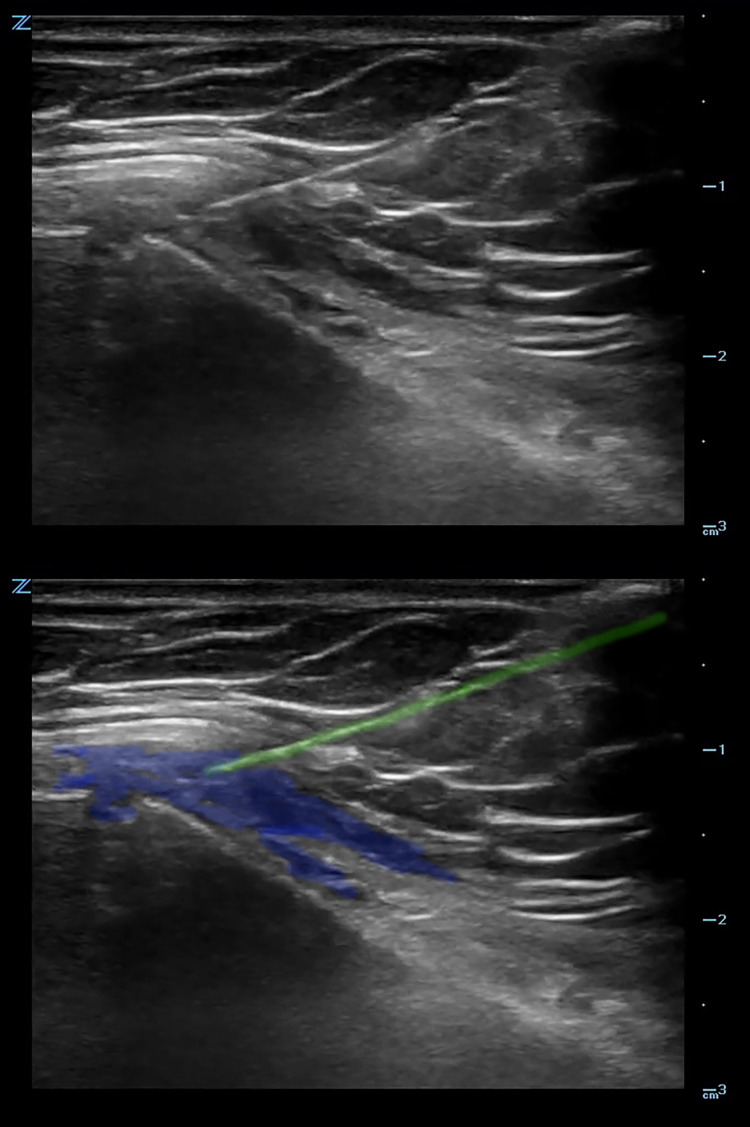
Ultrasound image after injection of local anesthetic into the hematoma. The needle (green) and the injected anesthetic (blue) are highlighted.
